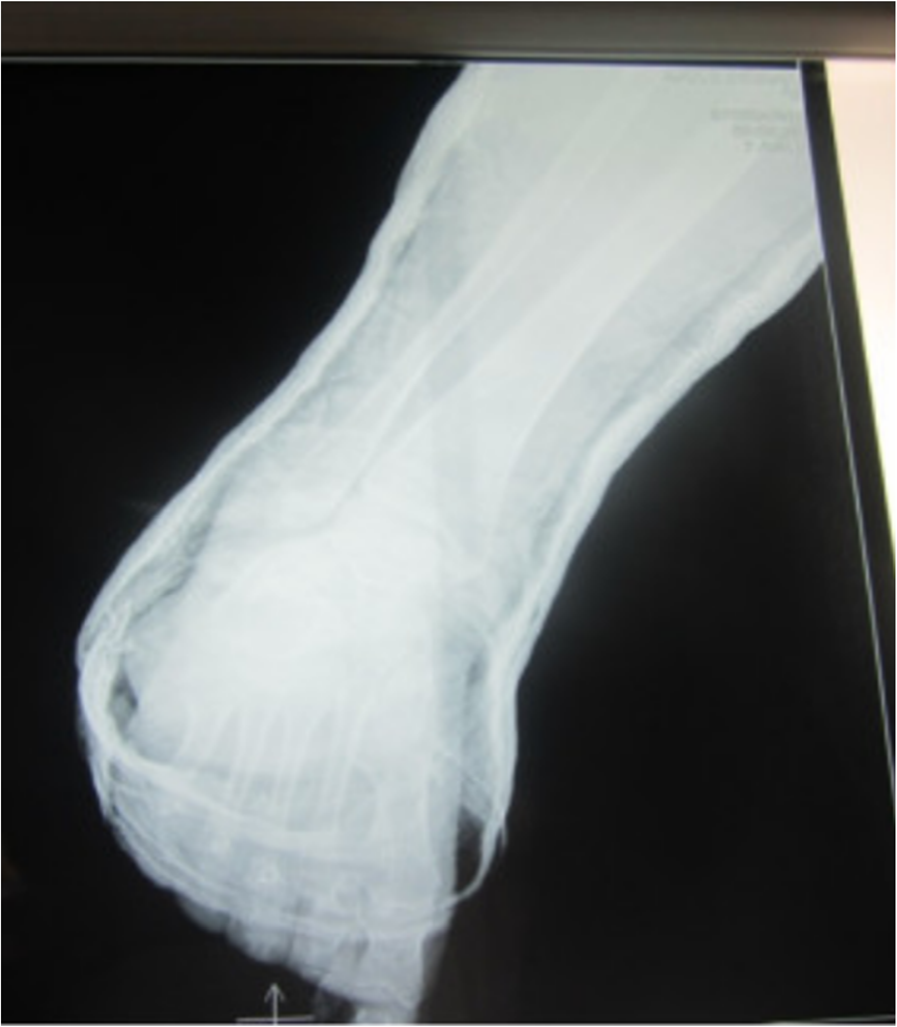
Control anteroposterior radiographs after reduction.
